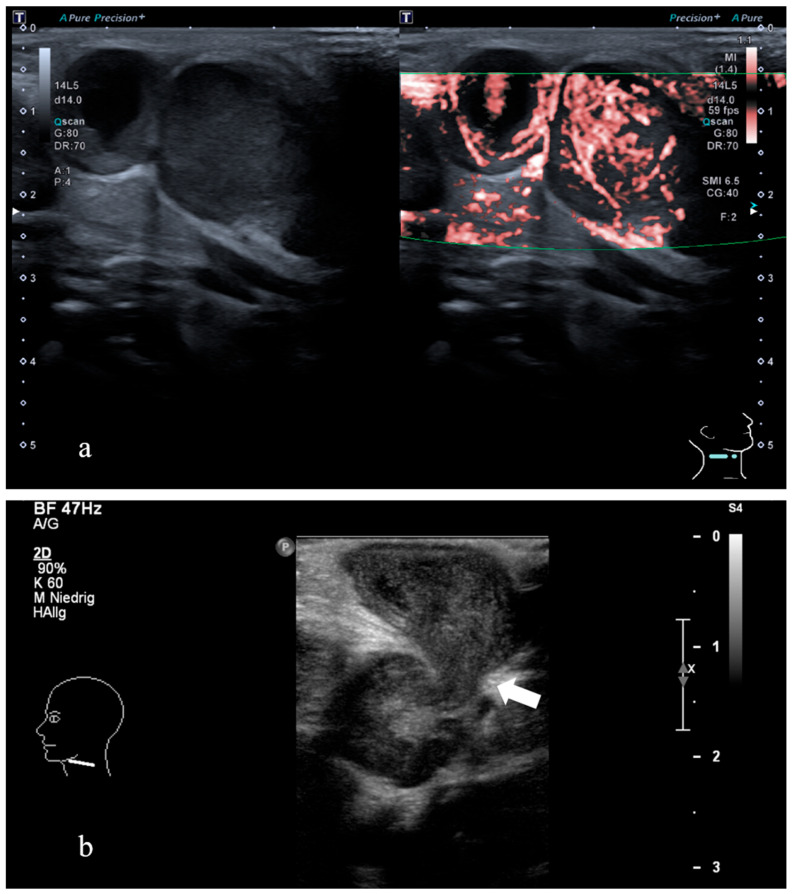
Ultrasound of a 2-year-old with cervical NTM lymphadenopathy. According to the clinical presentation, ultrasound showed a hypoechoic necrotic lymph node with irregular margins and irregular perfusion (a) and a fistulous tract consistent with skin perforation ((b), arrow) in level 2.
